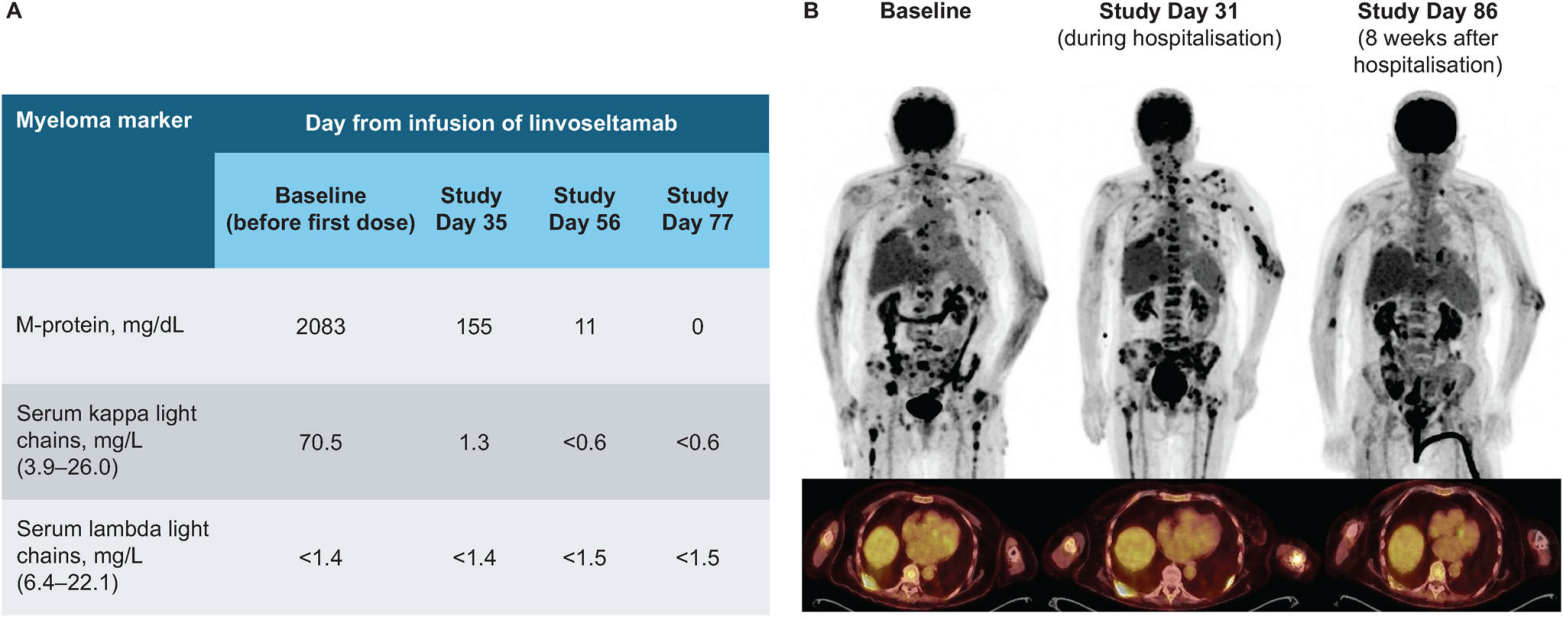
Disease and radiologic assessment of the patient before, during and after linvoseltamab treatment. (A) Myeloma protein markers from baseline (before the first dose of linvoseltamab) to study Day 77. For serum kappa and lambda light chains, the values in brackets represent the normal range, and the value beside “<” represents the lower limit of quantification for that assay. (B) PET/CT scan images prior to initiation of linvoseltamab (left), at the time of hospital admission (centre) and at approximately 8 weeks following hospitalisation (right). New FDG‐avid lesions and increased FDG avidity of existing lesions were observed at hospital admission, but many of these disappeared during the following 8 weeks. A new FDG‐avid lesion was identified in the left humerus at hospital admission, but had resolved at the scan 8 weeks post hospitalisation. FDG, 18F‐fluorodeoxyglucose; PET/CT, positron emission tomography/computed tomography.
